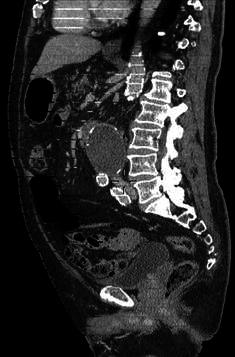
Computed tomography scan 1 month after endovascular abdominal aortic aneurysm repair. No signs of vertebral erosion are present
